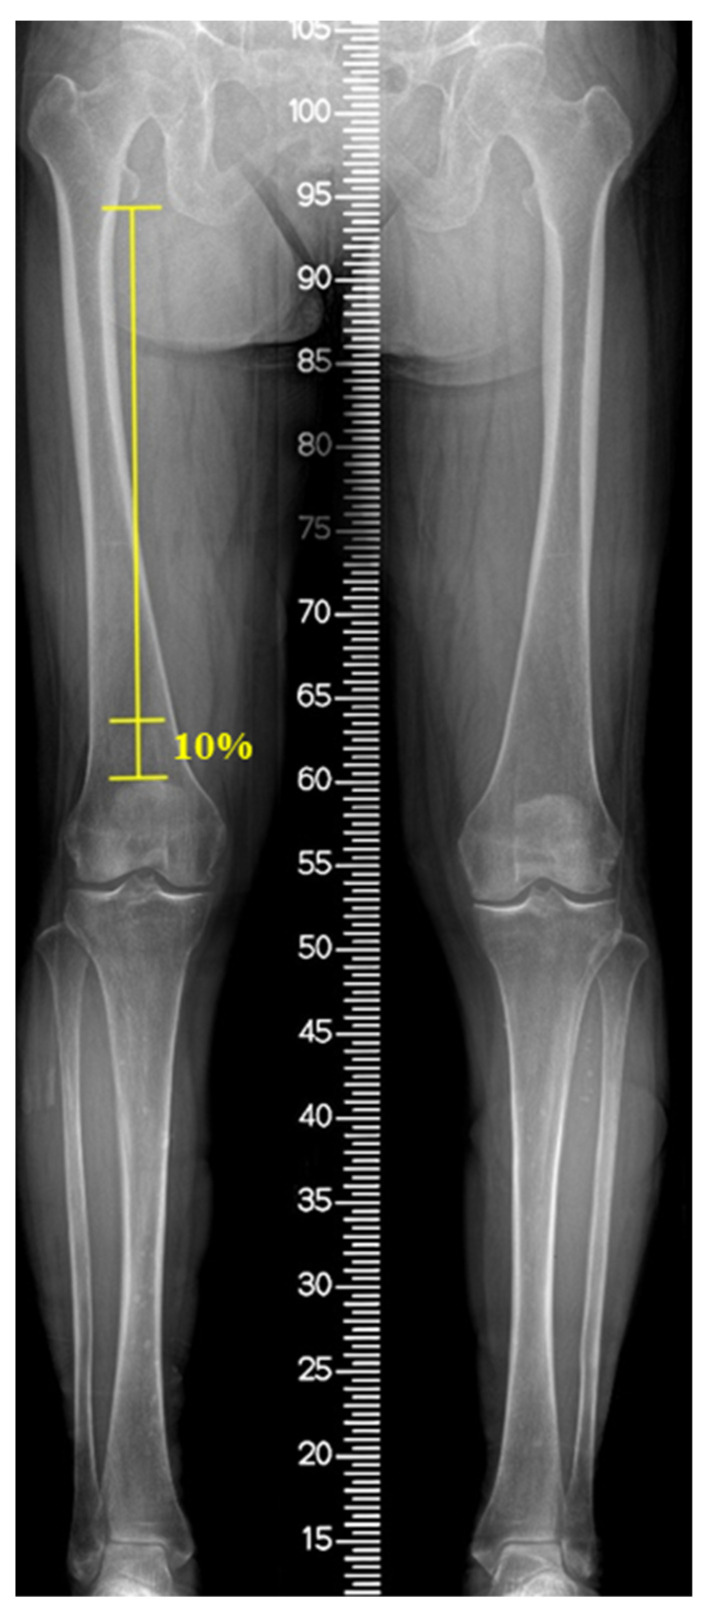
Measurement of the femoral length (FL), defined as the distance from the distal end of lesser trochanter to the superior surface of patella in full-length standing anteroposterior radiograph.
